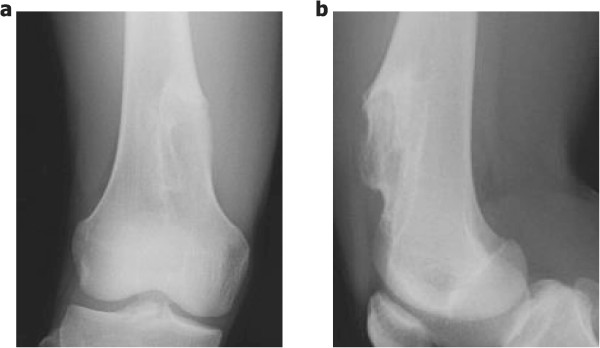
Preoperative plain radiographs. (a) Anteroposterior and (b) lateral radiographs showing cortical erosion, saucerization of the underlying cortex, and a rim of sclerosis on the anterior surface of the right distal femur.
